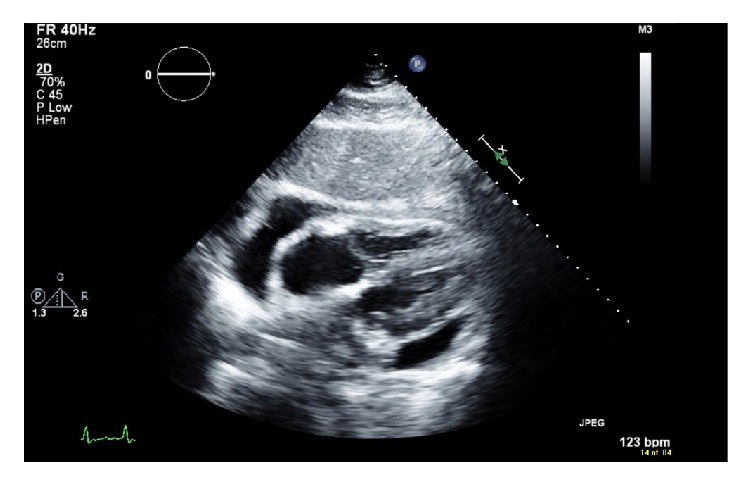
Subcostal view of the pericardial effusion on transthoracic echocardiogram.
